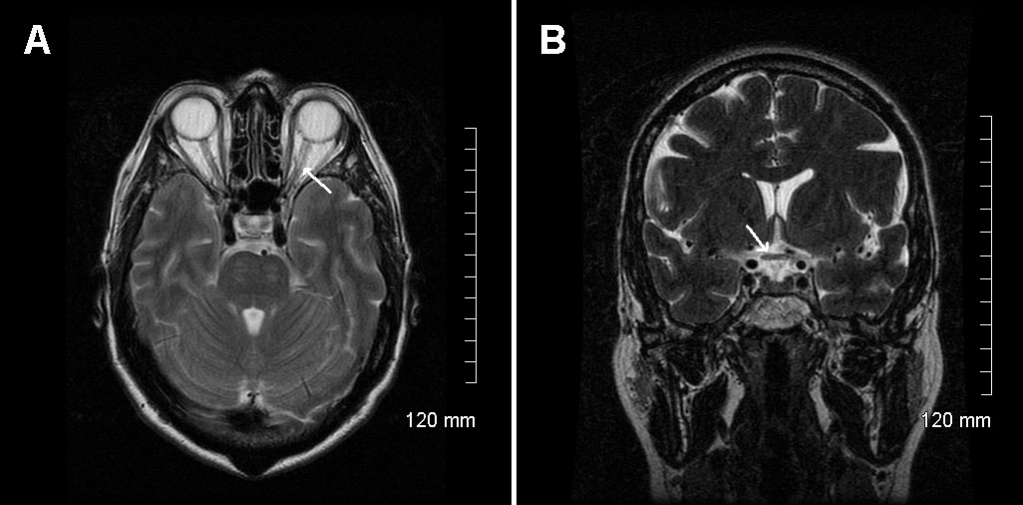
Patient's encephalic MRI. MRI of the optic nerve showed severe bilateral atrophy of the optic nerve from retina to the lateral geniculate nucleus, seen on horizontal MRI section (A), including atrophy of the optic chiasma, seen on coronal MRI section (B).
